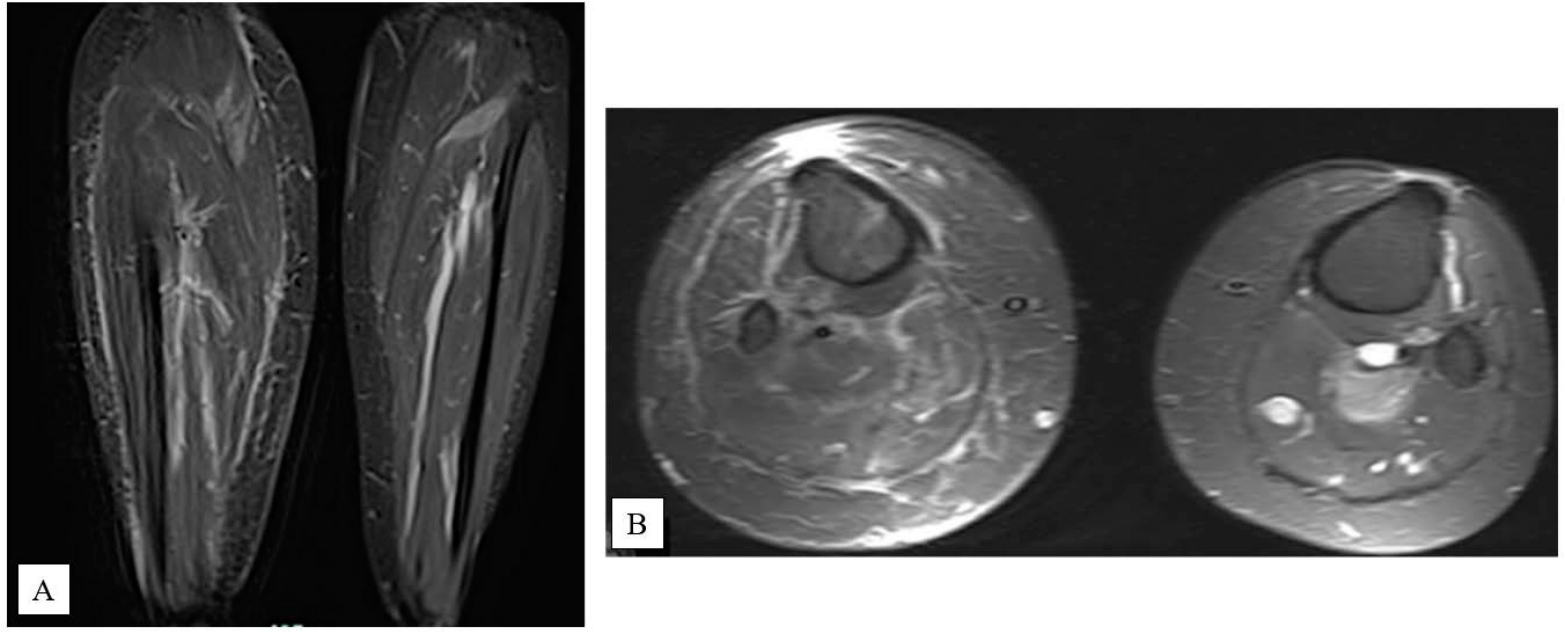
Coronal (A) and axial (B) T2-weighted magnetic resonance images of lower legs taken in December 2011. Note the right lower leg image showing a medial gastrocnemius mass measuring 1.6×2.1 cm (left arrow) and a second mass in the plantaris muscle measuring 1.9×3.1×2.3×2.6 cm (right arrow).
